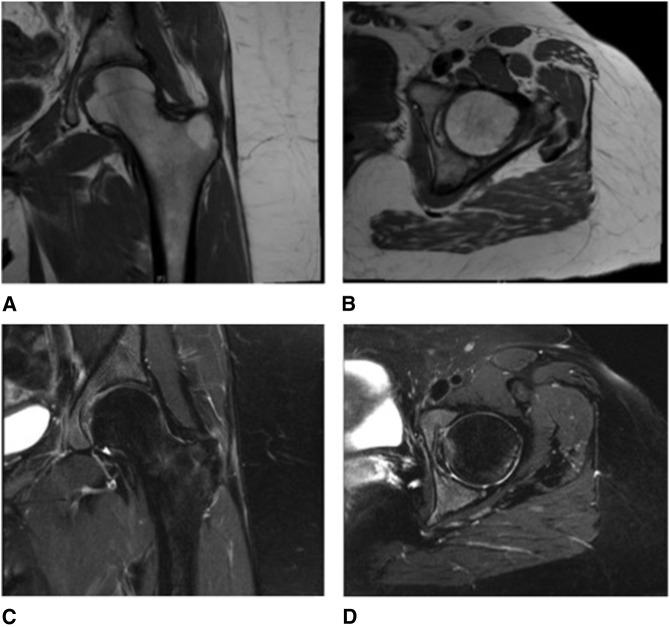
T1 coronal view (A), T1 axial view (B), T2 coronal view (C), and T2 axial view (D) of left hip MRI performed 6 months postpartum, showing near complete resolution of osteonecrosis of left femoral head.
